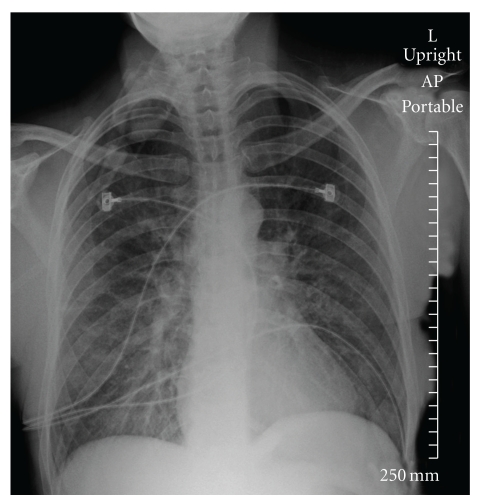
Case 2 chest X-ray at time 1744. Abnormal chest X-ray taken on admission to the emergency room. shows bilateral airspace with ground glass predominately on the lower right side. The image shows no Kerley B lines, vascular redistribution, or pleural effusions. Heart size appears normal.
